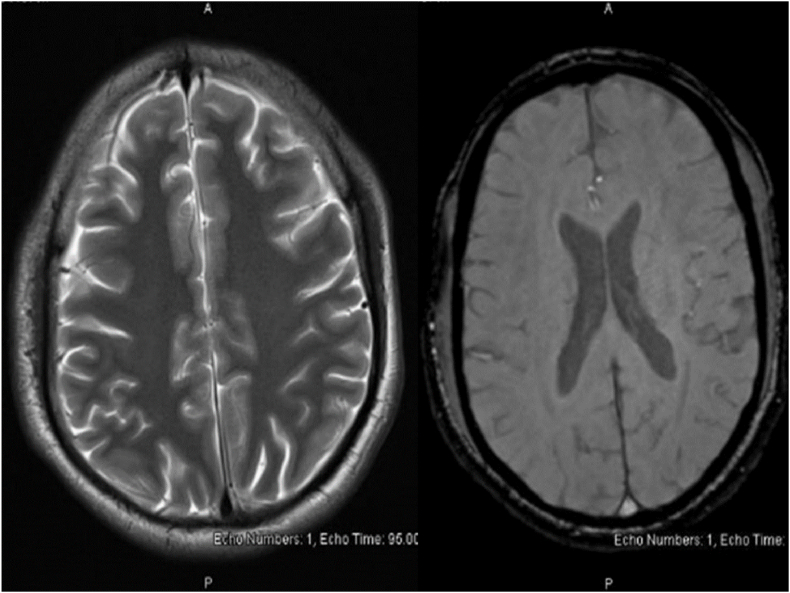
MRI of the brain revealed no abnormality.
